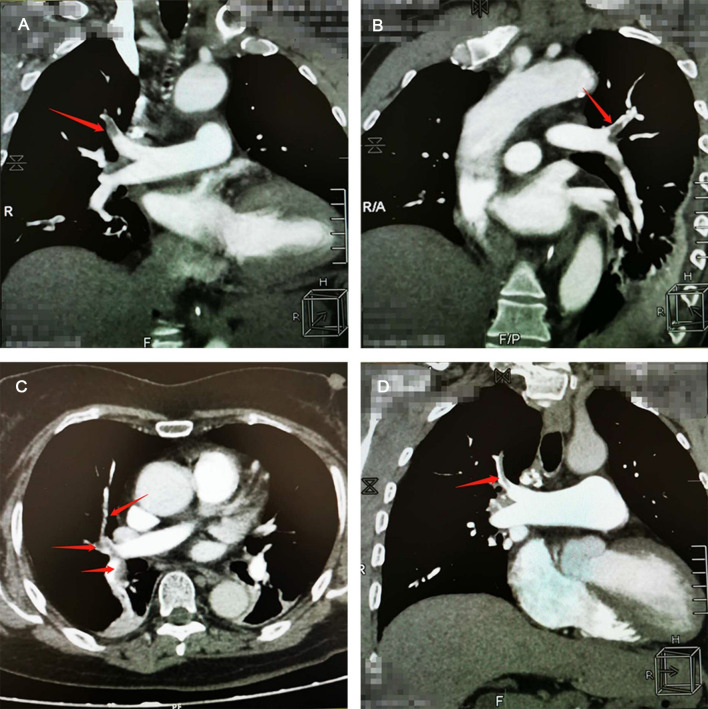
Representative computed tomographic pulmonary arteriography (CTPA) images of pulmonary embolism (PE). (A) CTPA shows filling defects in the right upper pulmonary artery (red arrow). (B) CTPA shows filling defects in the left upper pulmonary artery (red arrow). (C) CTPA shows filling defects in the right upper pulmonary artery, right middle pulmonary artery, and right lower pulmonary artery (red arrow). (D) CTPA shows filling defects in the right upper pulmonary artery (red arrow).
